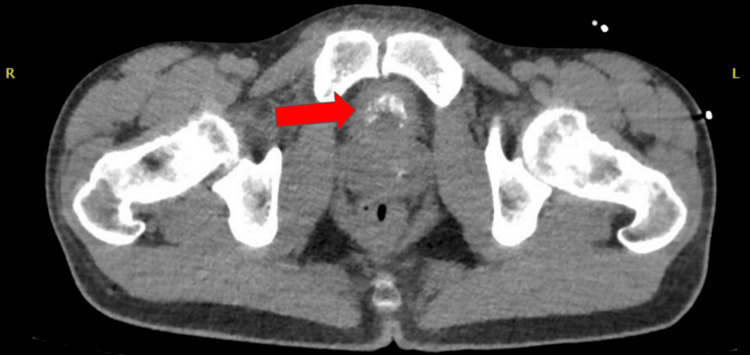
Axial CT (renal tract protocol) demonstrating prostatic calcification (arrow).
